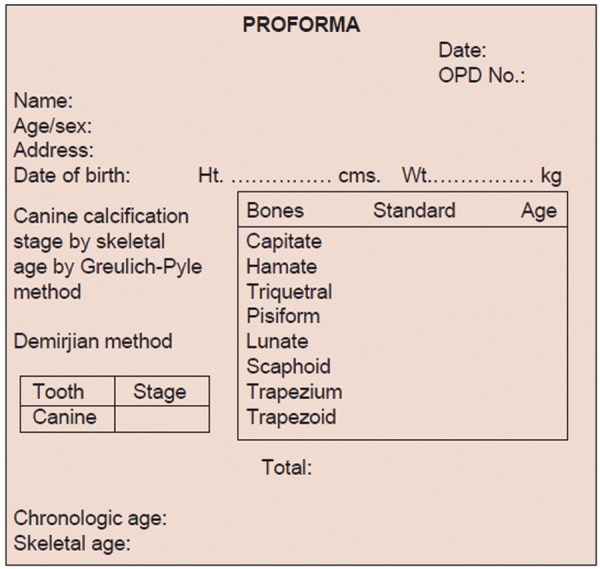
Radiographs for each subject were taken in the department of oral medicine and radiology. All the exposed films were developed, fixed and dried.
